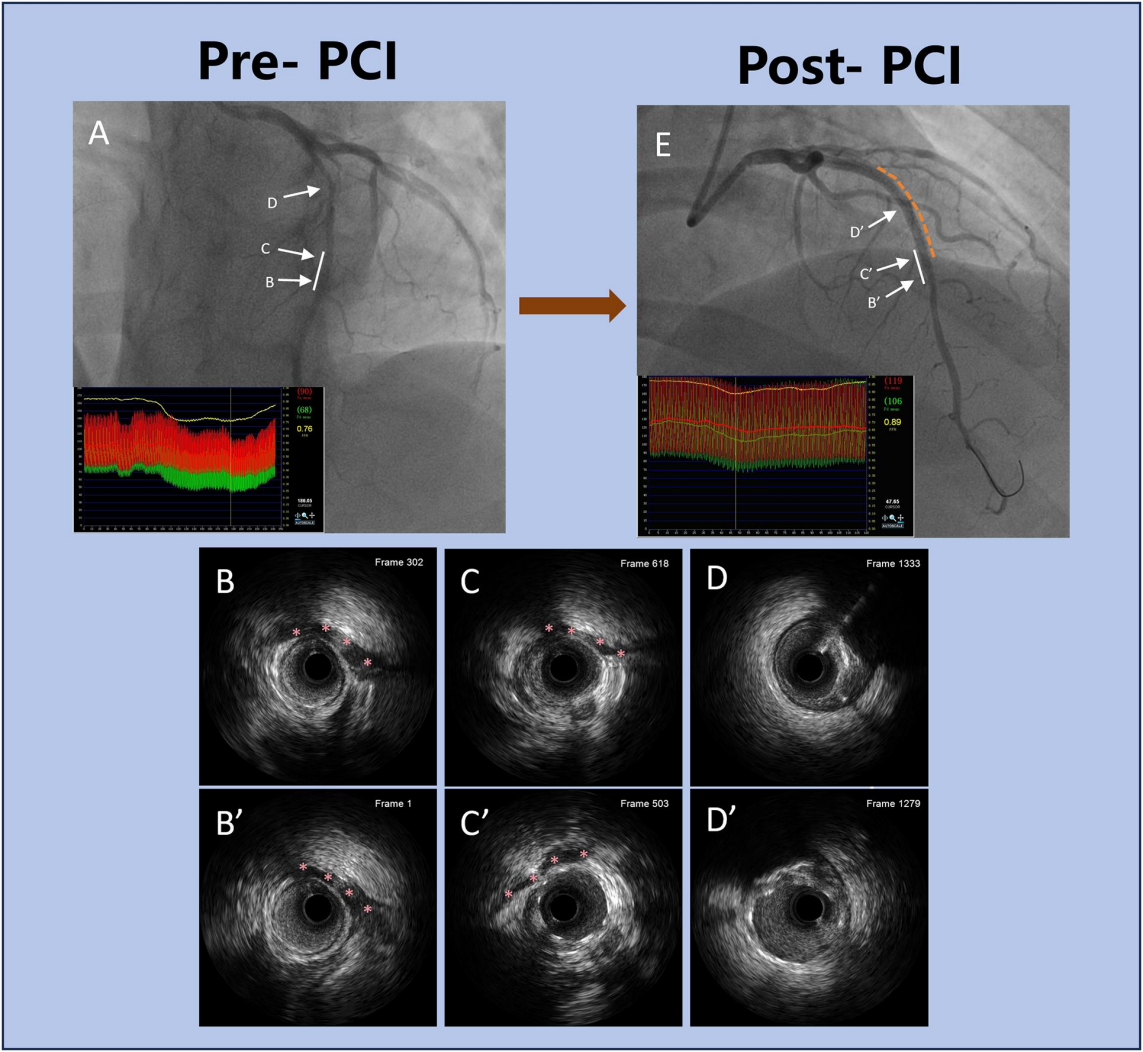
Representative clinical case of PCI in a patient with proximal LAD stenosis and MB. (A) Pre-PCI coronary angiography showing moderate proximal LAD stenosis adjacent to a MB. The white solid line indicates the MB segment. The co-registered FFR tracing reveals a nadir value of 0.76 distal to the MB, indicating functionally significant ischemia. (B–D) Corresponding pre-PCI IVUS images at levels (B–D). Red asterisks in (B,C) mark the MB segment identified on IVUS, showing systolic compression and plaque burden. (E) Post-PCI coronary angiography after DES deployment. The white solid line again marks the MB segment, and the yellow dashed line outlines the stented portion of the vessel. FFR improved to 0.89, confirming successful revascularization with improved coronary physiology. (B’–D’) Post-PCI IVUS images at corresponding levels B’–D’. Red asterisks in B’ and C’ indicate the MB segment, showing adequate stent expansion and apposition outside the bridged area, with no evidence of malapposition or dissection. This case highlights the integration of anatomical imaging (angiography, IVUS) and FFR to guide optimal PCI strategy, particularly in lesions involving MB. PCI, percutaneous coronary intervention; LAD, left anterior descending artery; MB, myocardial bridging; FFR, fractional flow reserve; IVUS, intravascular ultrasound; DES, drug-eluting stent.
